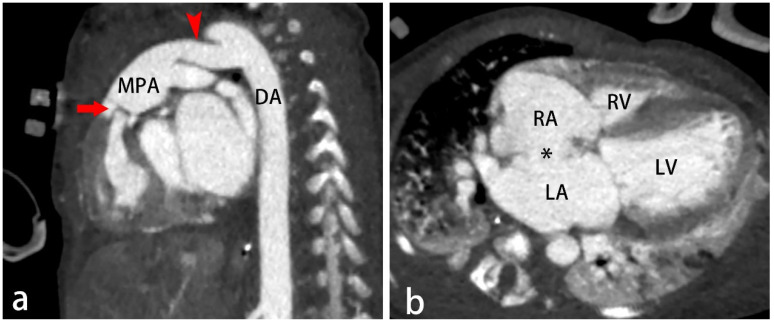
A 10-day-old girl with pulmonary atresia with intact ventricular septum. Oblique sagittal maximum intensity projection (MIP) CT angiography shows pulmonary valve atresia ((a), red arrow) and dilated main pulmonary artery, which is supplied by the aorta via a patent ductus arteriosus ((a), red arrowhead). The CT angiography four-chamber view shows an intact ventricular septum and an atrial septal defect ((b), black asterisk). LV, left ventricle; LA, left atrium; RV, right ventricle; RA, right atrium; DA, descending aorta; MPA, main pulmonary artery.
